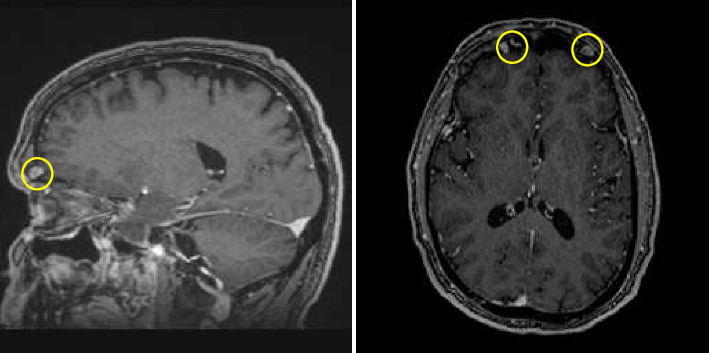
MRI of the brain in sagittal and axial views. The lesions are outlined with yellow circles.
